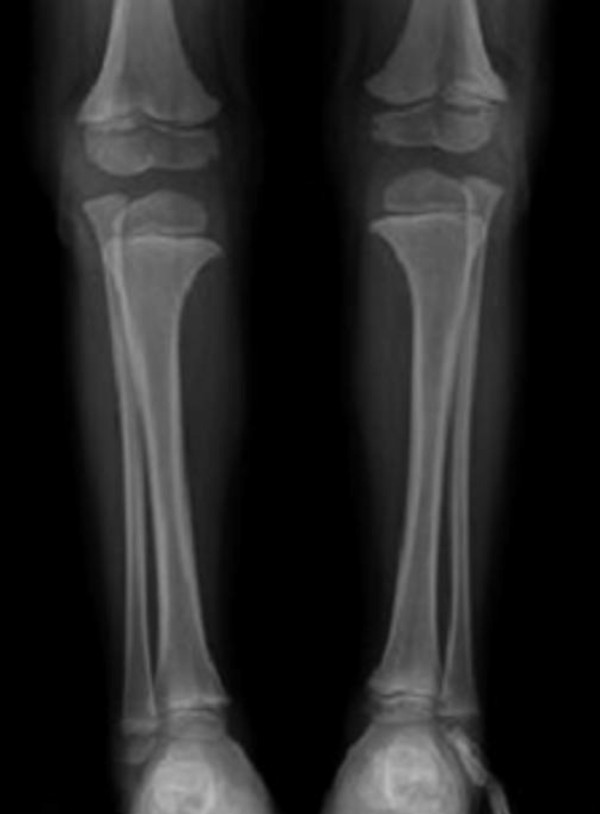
Anteroposterior radiograph of the lower limbs showed unduly long fibulae causing effectively medial tibial torsion and a tongue like projection of the medial metaphyses of the proximal tibia. Significant bilateral tibial dysplasia was apparent.
